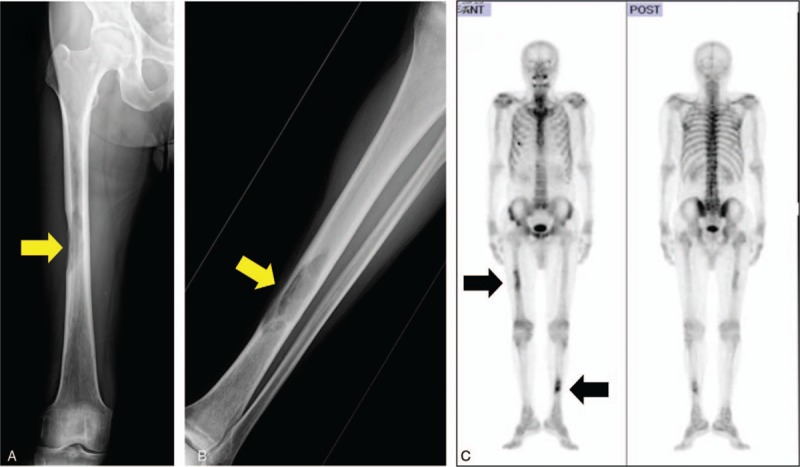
X-ray and bone scintigraphy after dosing with pazopanib. X-ray showing a radiolucent shadow at the right femur diaphysis (A, arrow) and left tibia diaphysis (B, arrow). (C) Bone scintigraphy showing metastases at the right femur diaphysis and left tibia diaphysis (arrows).
